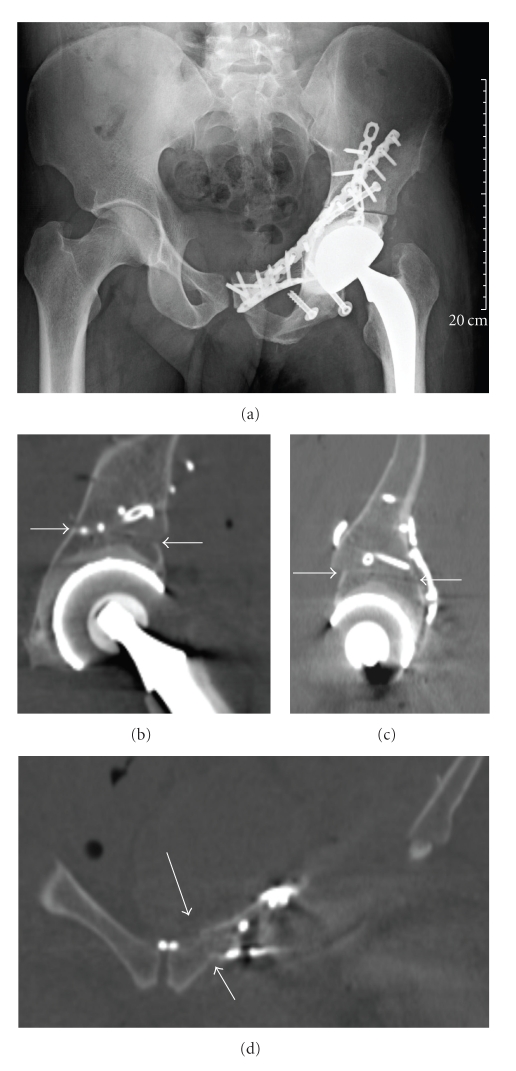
(a) Postoperative radiograph of the pelvis showing the reconstruction. (b) CT with coronal reconstruction showing the host-graft junction (white arrows) in the supraacetabular area. (c) Sagittal reconstruction of the supraacetabular junction (white arrows). (d) Coronal reconstruction of the pubic junction (white arrows).
